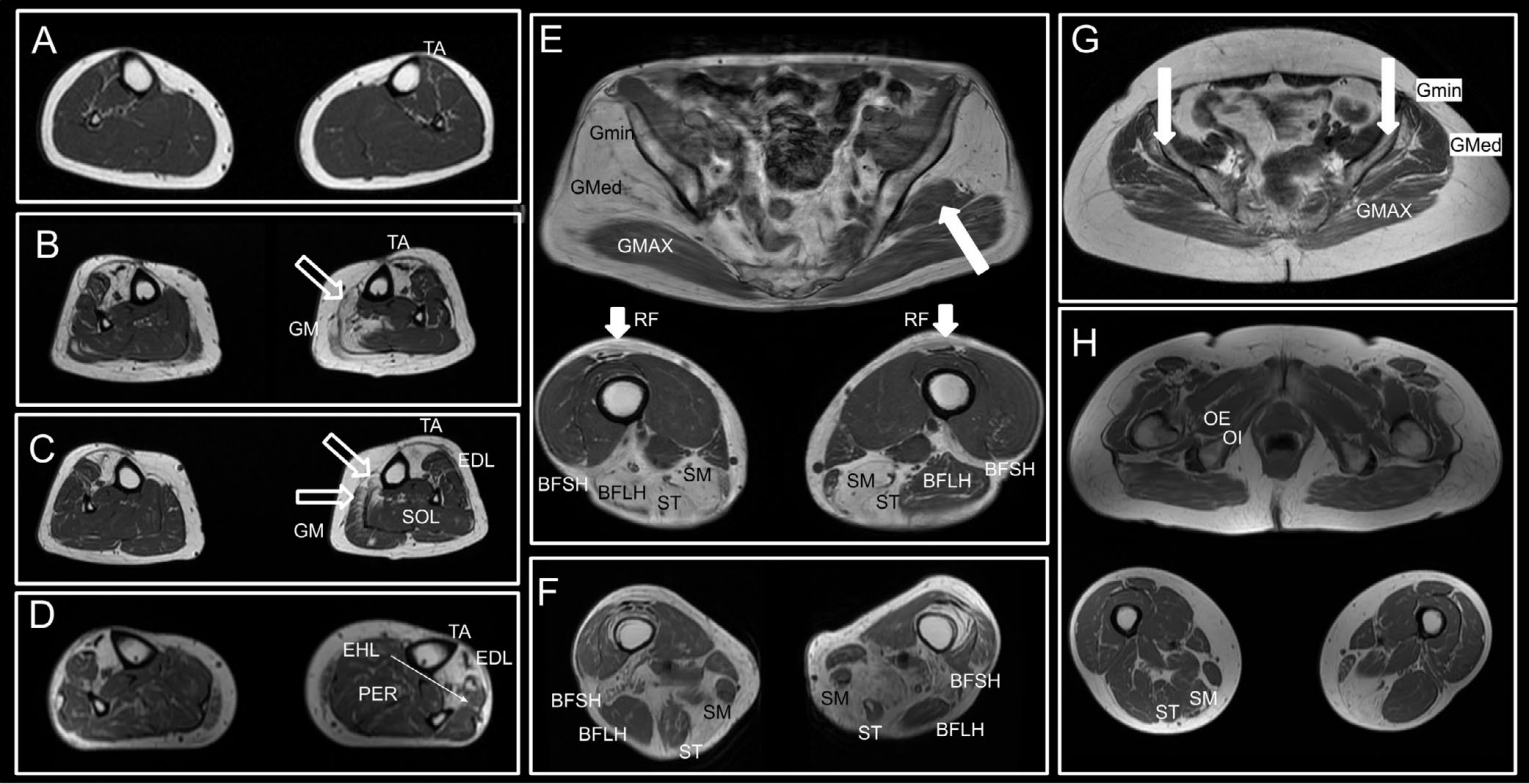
Selected MR images from different patients with TMD. (A–D) Axial images from leg muscles. Tibialis anterior (TA) is early involved, even in patients with minimal fat replacement (A). Generally tibialis anterior is severely involved (B–D). Extensor digitorum longus (EDL) is assymetrically involved. Some patients have assymetric and non‐homogeneous involvement of soleus (SOL) and gastrocnemius (GM). Peroneal muscles are mostly preserved (E–H). Axial images from pelvis and thigh of four different patients. Notice the asymmetry and very selective involvement of some muscles. The involvement of gluteus minimus (Gmin) that could be mild (G) or severe (E). Gluteus medium (GMed) could also be involved (E). Semimembranosus (SM), semitendinosus (ST) and biceps femoris (BFSH‐short head and BFLH—long head) are the other most commonly involved muscle outside the leg. Some patients may have severe atrophy of rectus femoris (RF). Obturator externus (OE) and internus (OI) are preserved muscles (H‐pelvis).
